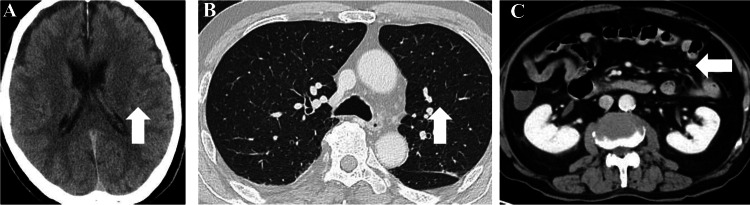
CT scans on illness day four. (A) Non-contrast CT scan of the head. (B) Contrast-enhanced CT scan of the chest. (C) Contrast-enhanced CT scan of the abdomen and pelvis. No significant abnormalities were observed in any of the scans. The arrows indicate typical structures to show the absence of pathological changes.
